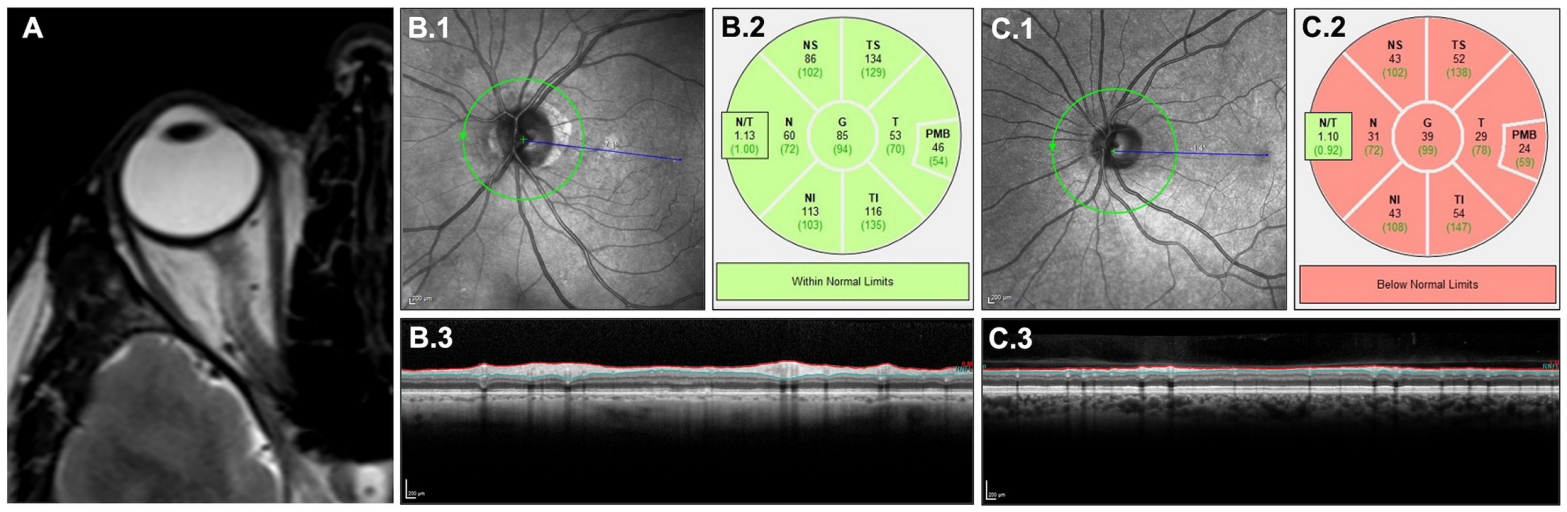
Clinical imaging of retina and optic nerve. T2-weighted MRI of the optic nerve (A) showing a longitudinal lesion with edema. OCT quantifying retinal neuroaxonal content measured by pRNFL around the optic nerve head in a retina without a history of ON (B) and with a history of ON (C) in MOGAD: scanning laser ophthalmoscopy (B.1, C.1), color-coded comparison with a healthy control cohort (B.2, C.2) and cross-sectional B-scans showing pRNFL atrophy in (C.3) compared with (B.3). MOGAD, myelin oligodendrocyte glycoprotein antibody associated disease; MRI, magnetic resonance imaging; OCT, optical coherence tomography; ON, optic neuritis; pRNFL, peripapillary retinal nerve fiber layer.
